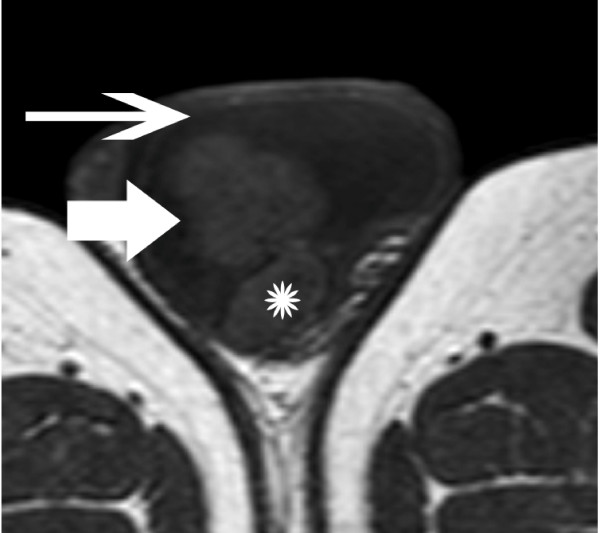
Transverse T1-weighted image depicts a multilobular left scrotal mass (arrow), located in the paratesticular space. The lesion had similar signal intensity, when compared to the normal testicular parenchyma (asterisk). Left hydrocele (long arrow).
